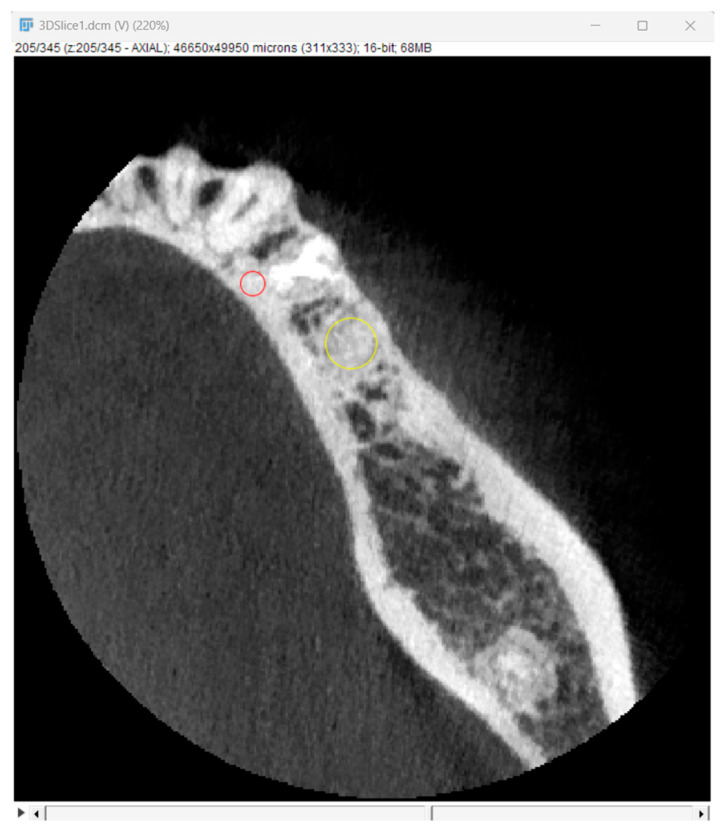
Radiographic optical density of bone measured using ImageJ software. The yellow circle represents the ROI where the measurements were taken, and the red circle represents the positioning pin used for orientation during the measurements.
